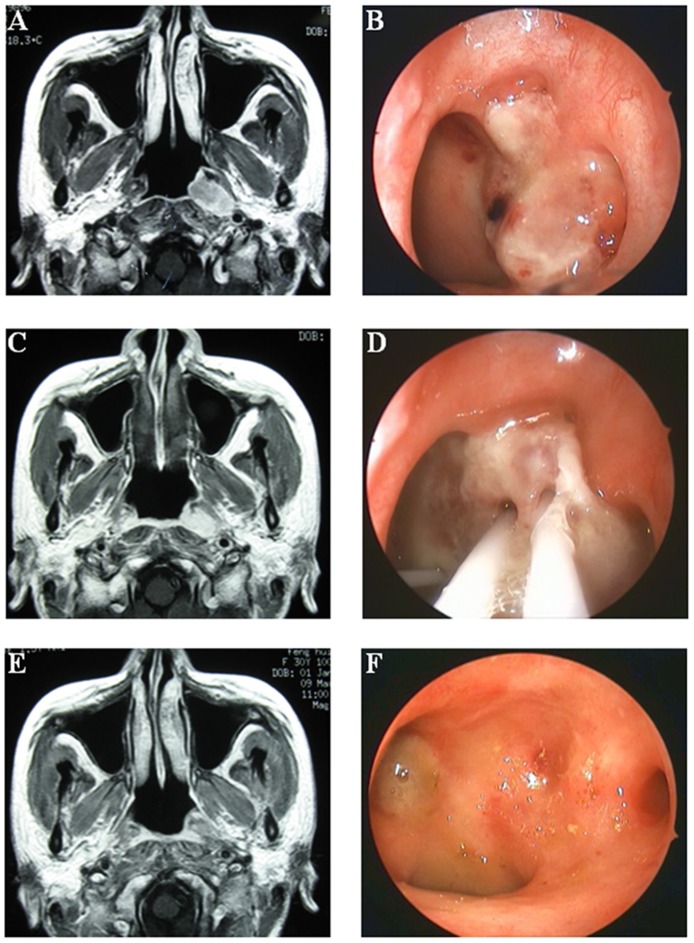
The representative images prior to, during and after the IMBT boost for T2b NPC. MRI view (A) and endoscopic image (B) of the nasopharyngeal carcinoma prior to the external beam radiotherapy. MRI view (C) and endoscopic image (D) of the residual nasopharyngeal carcinoma after the external beam radiotherapy. MRI view (E) and endoscopic image (F) of the nasopharyngeal after the external beam radiotherapy and IMBT boost radiation.
